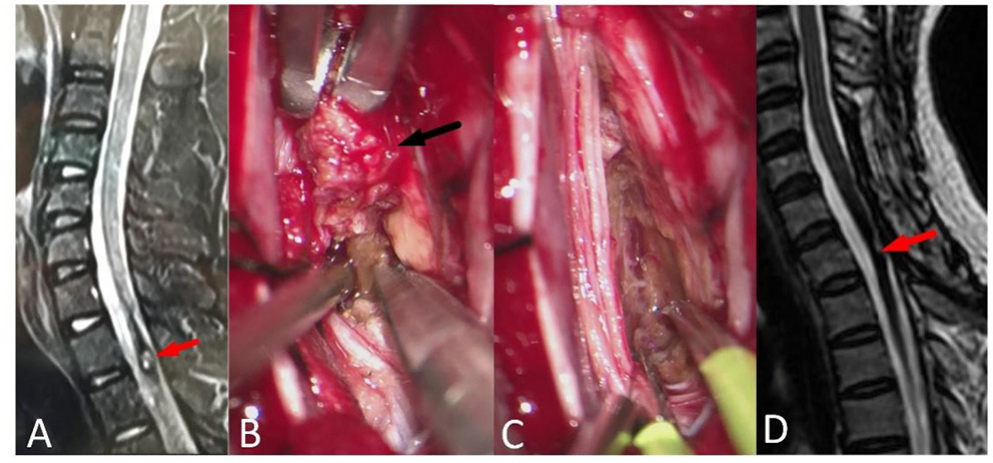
A 15-year-old female with walking difficulty for 2 months and aggravating for 5 days (mALs = 12 points, severe disorder, Type B1), was treated by ERS. (A) Preoperative spinal MRI examination revealed ISCH in T1-T2 (red arrow). (B,C) The lesion was resected in pieces (black arrow) by posterior central approach. (D) MRI reexamination showed no residual or recurrence at 2 years after surgery and the mAS was 8 points at the last follow-up.
